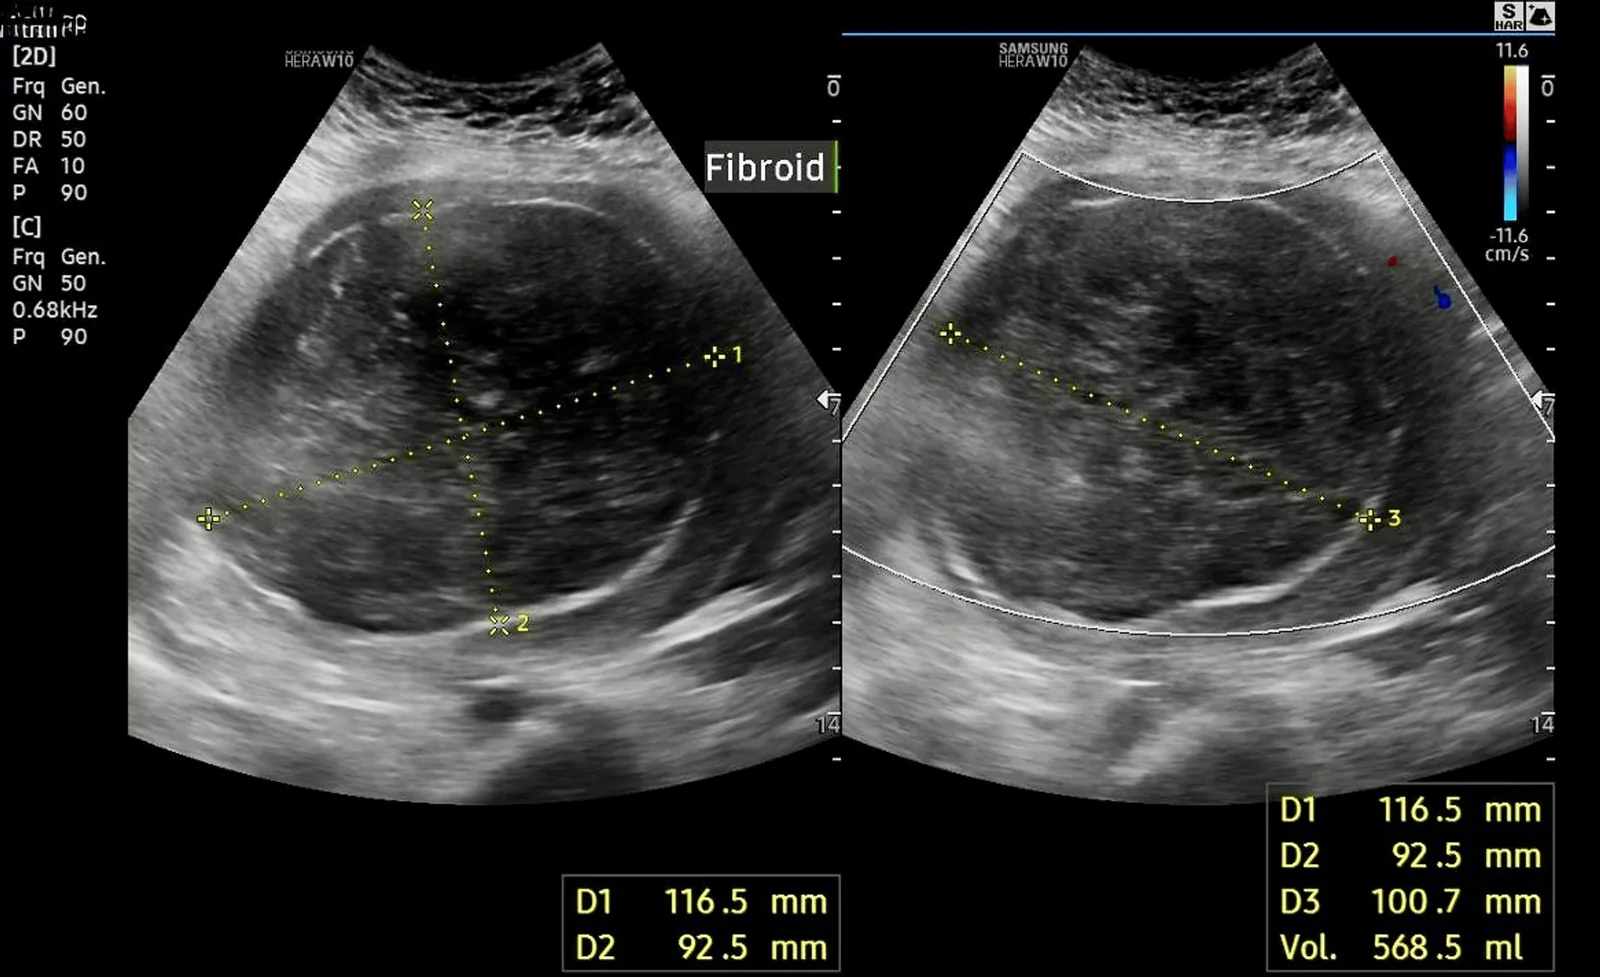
Three-month postpartum transabdominal ultrasound demonstrating a large submucosal fibroid measuring 116.5 × 92.5 × 100.7 mm, with significant distortion of the endometrial cavity. No clear sonographic evidence of retained products of conception was identified, and the endometrial stripe could not be reliably measured due to cavity distortion by the fibroid.
